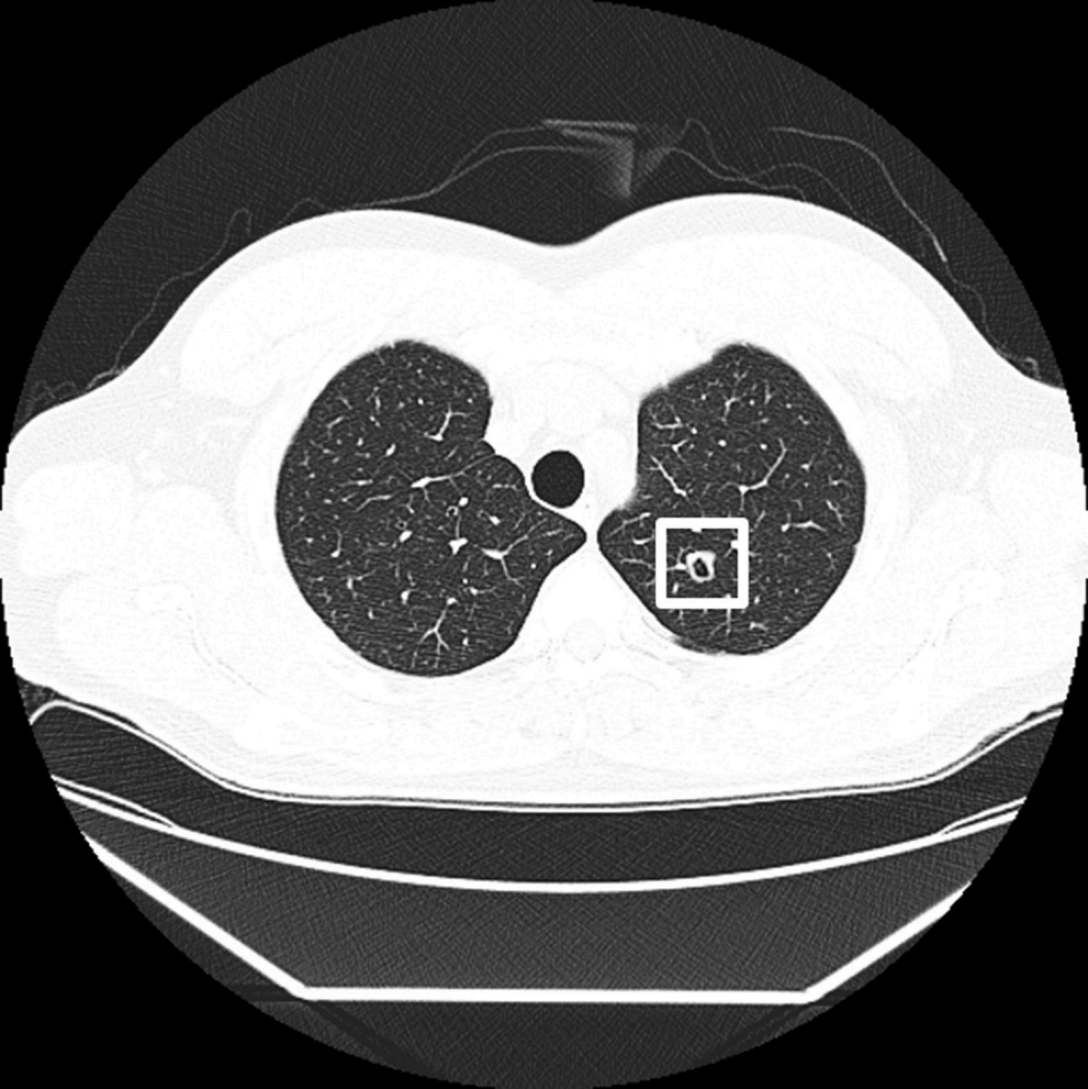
An example of the background CT image and a target lesion image used in the experiments. The target lesion is marked by a white frame, which was not presented in the experiment, in this figure.
